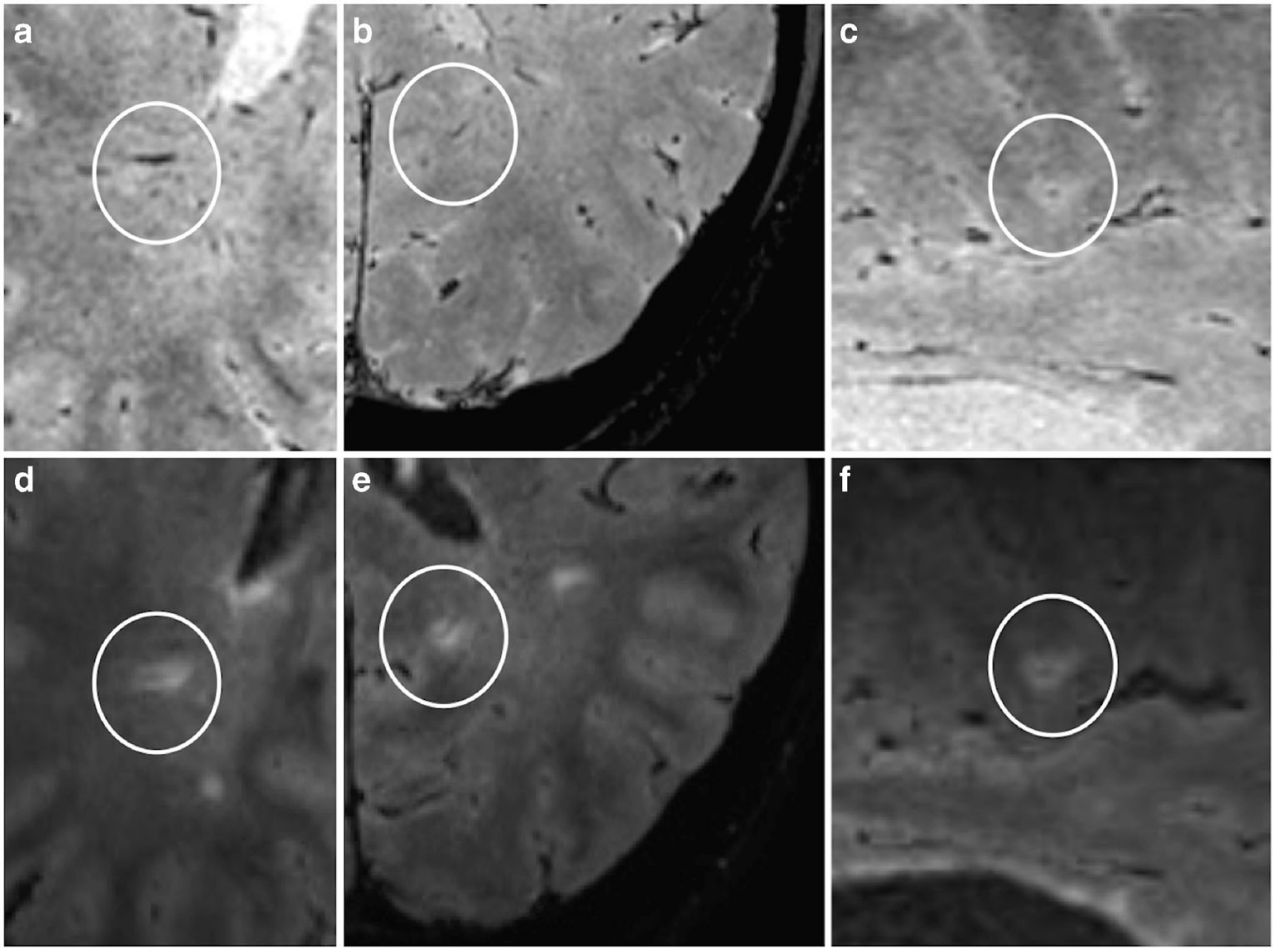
T_2_* versus FLAIR*. (a–c) Partial T_2_* slices from three patients with multiple sclerosis (MS): (a) axial, (b) axial and (c) coronal. White circle indicates vein, although lesions not clearly identified. (d–f) Corresponding partial FLAIR* slices at same level. White circle indicates white matter lesion around the same vein
